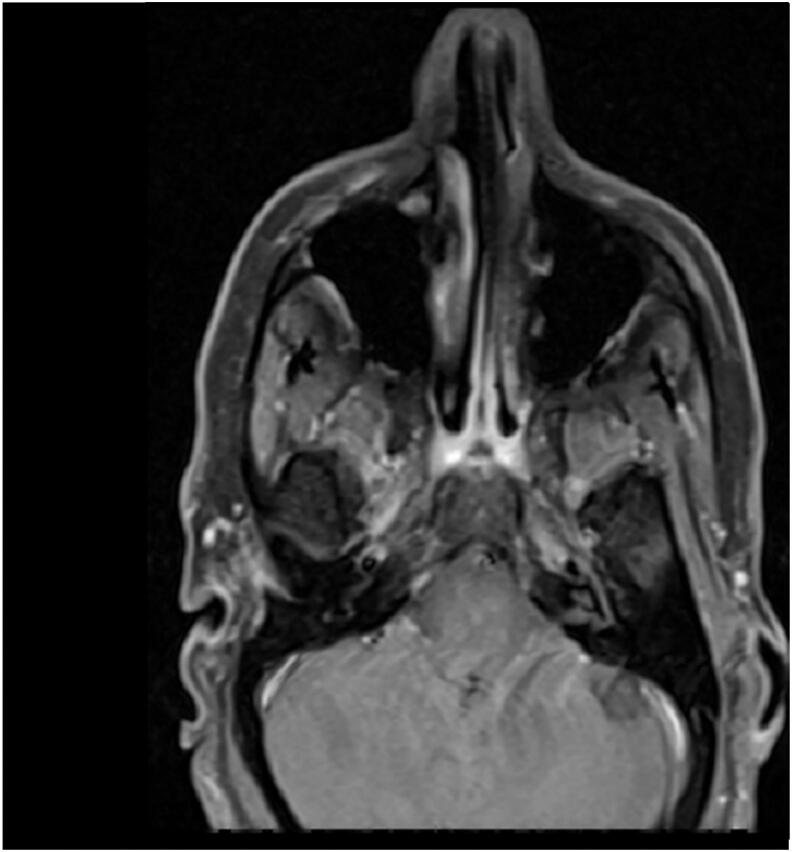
MRI of Right Maxillary sinus involved by Tumor.
